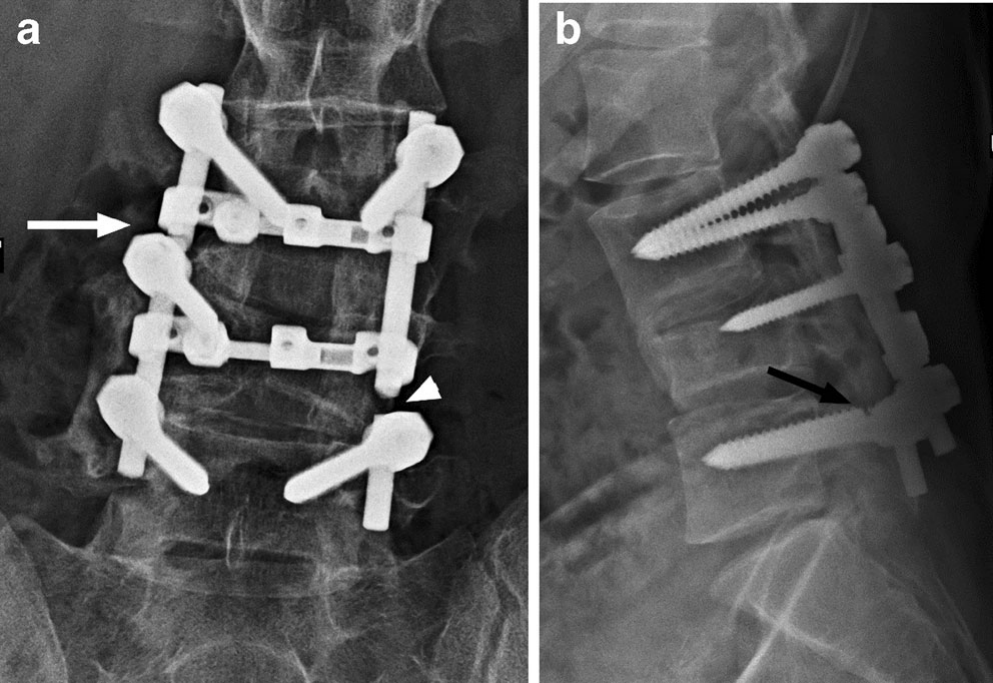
Hardware complication: a 24-year-old male presents with back pain 24 months after posterior segmental instrumentation and fusion of L3 to L5 for SCI because of a crush injury. a Antero-posterior radiograph shows disruption and distraction of the left fusion rod (*white arrowhead*). Failure of the right fusion rod is less conspicuous (*white arrow*). b The lateral radiograph demonstrates a break in the L5 pedicle screw (*black arrow*). Follow-up radiographs have to be carefully scrutinised for the presence of hardware failure and comparison with priors is essential
